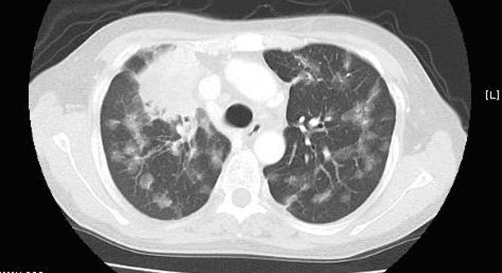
Chest CT showing a partial response of the primary site and multiple metastases of the bilateral lungs.
